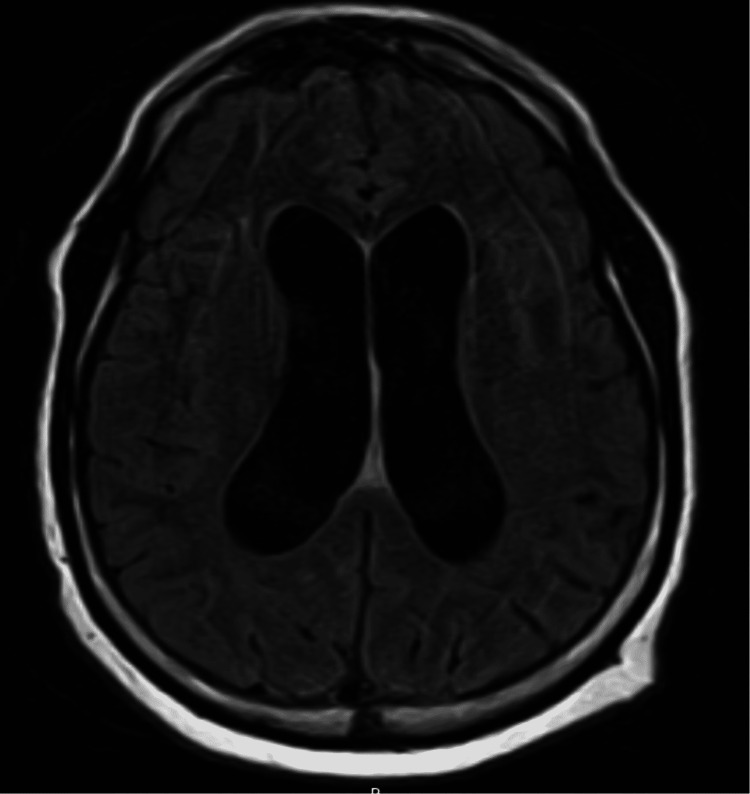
MRI of the brain demonstrating moderate ventricular dilation concerning for communicating hydrocephalus
